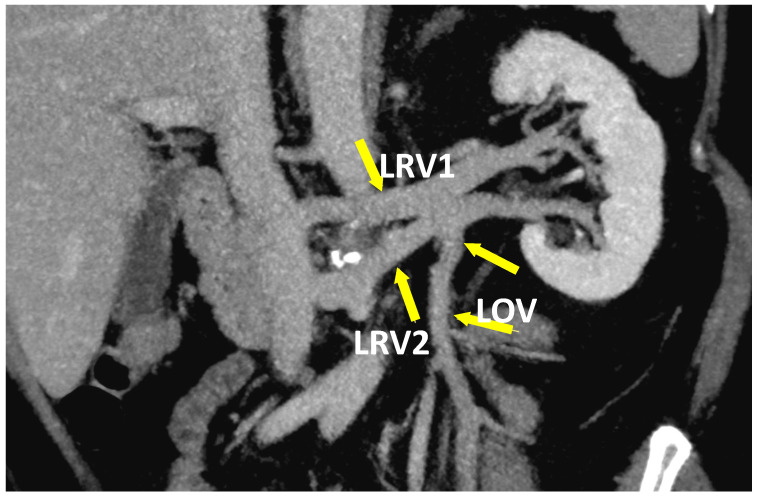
The coronal plane of CT-V scan in patient with duplicated LRV (LRV1 and LRV2). Blood from LOV is drained into LRV2.
